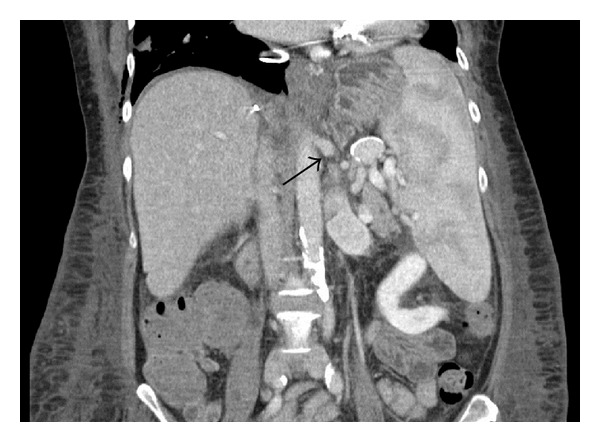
Postoperative CT imaging (day 5) after the ligation of the left renal vein. The arrow shows the point of ligation.
